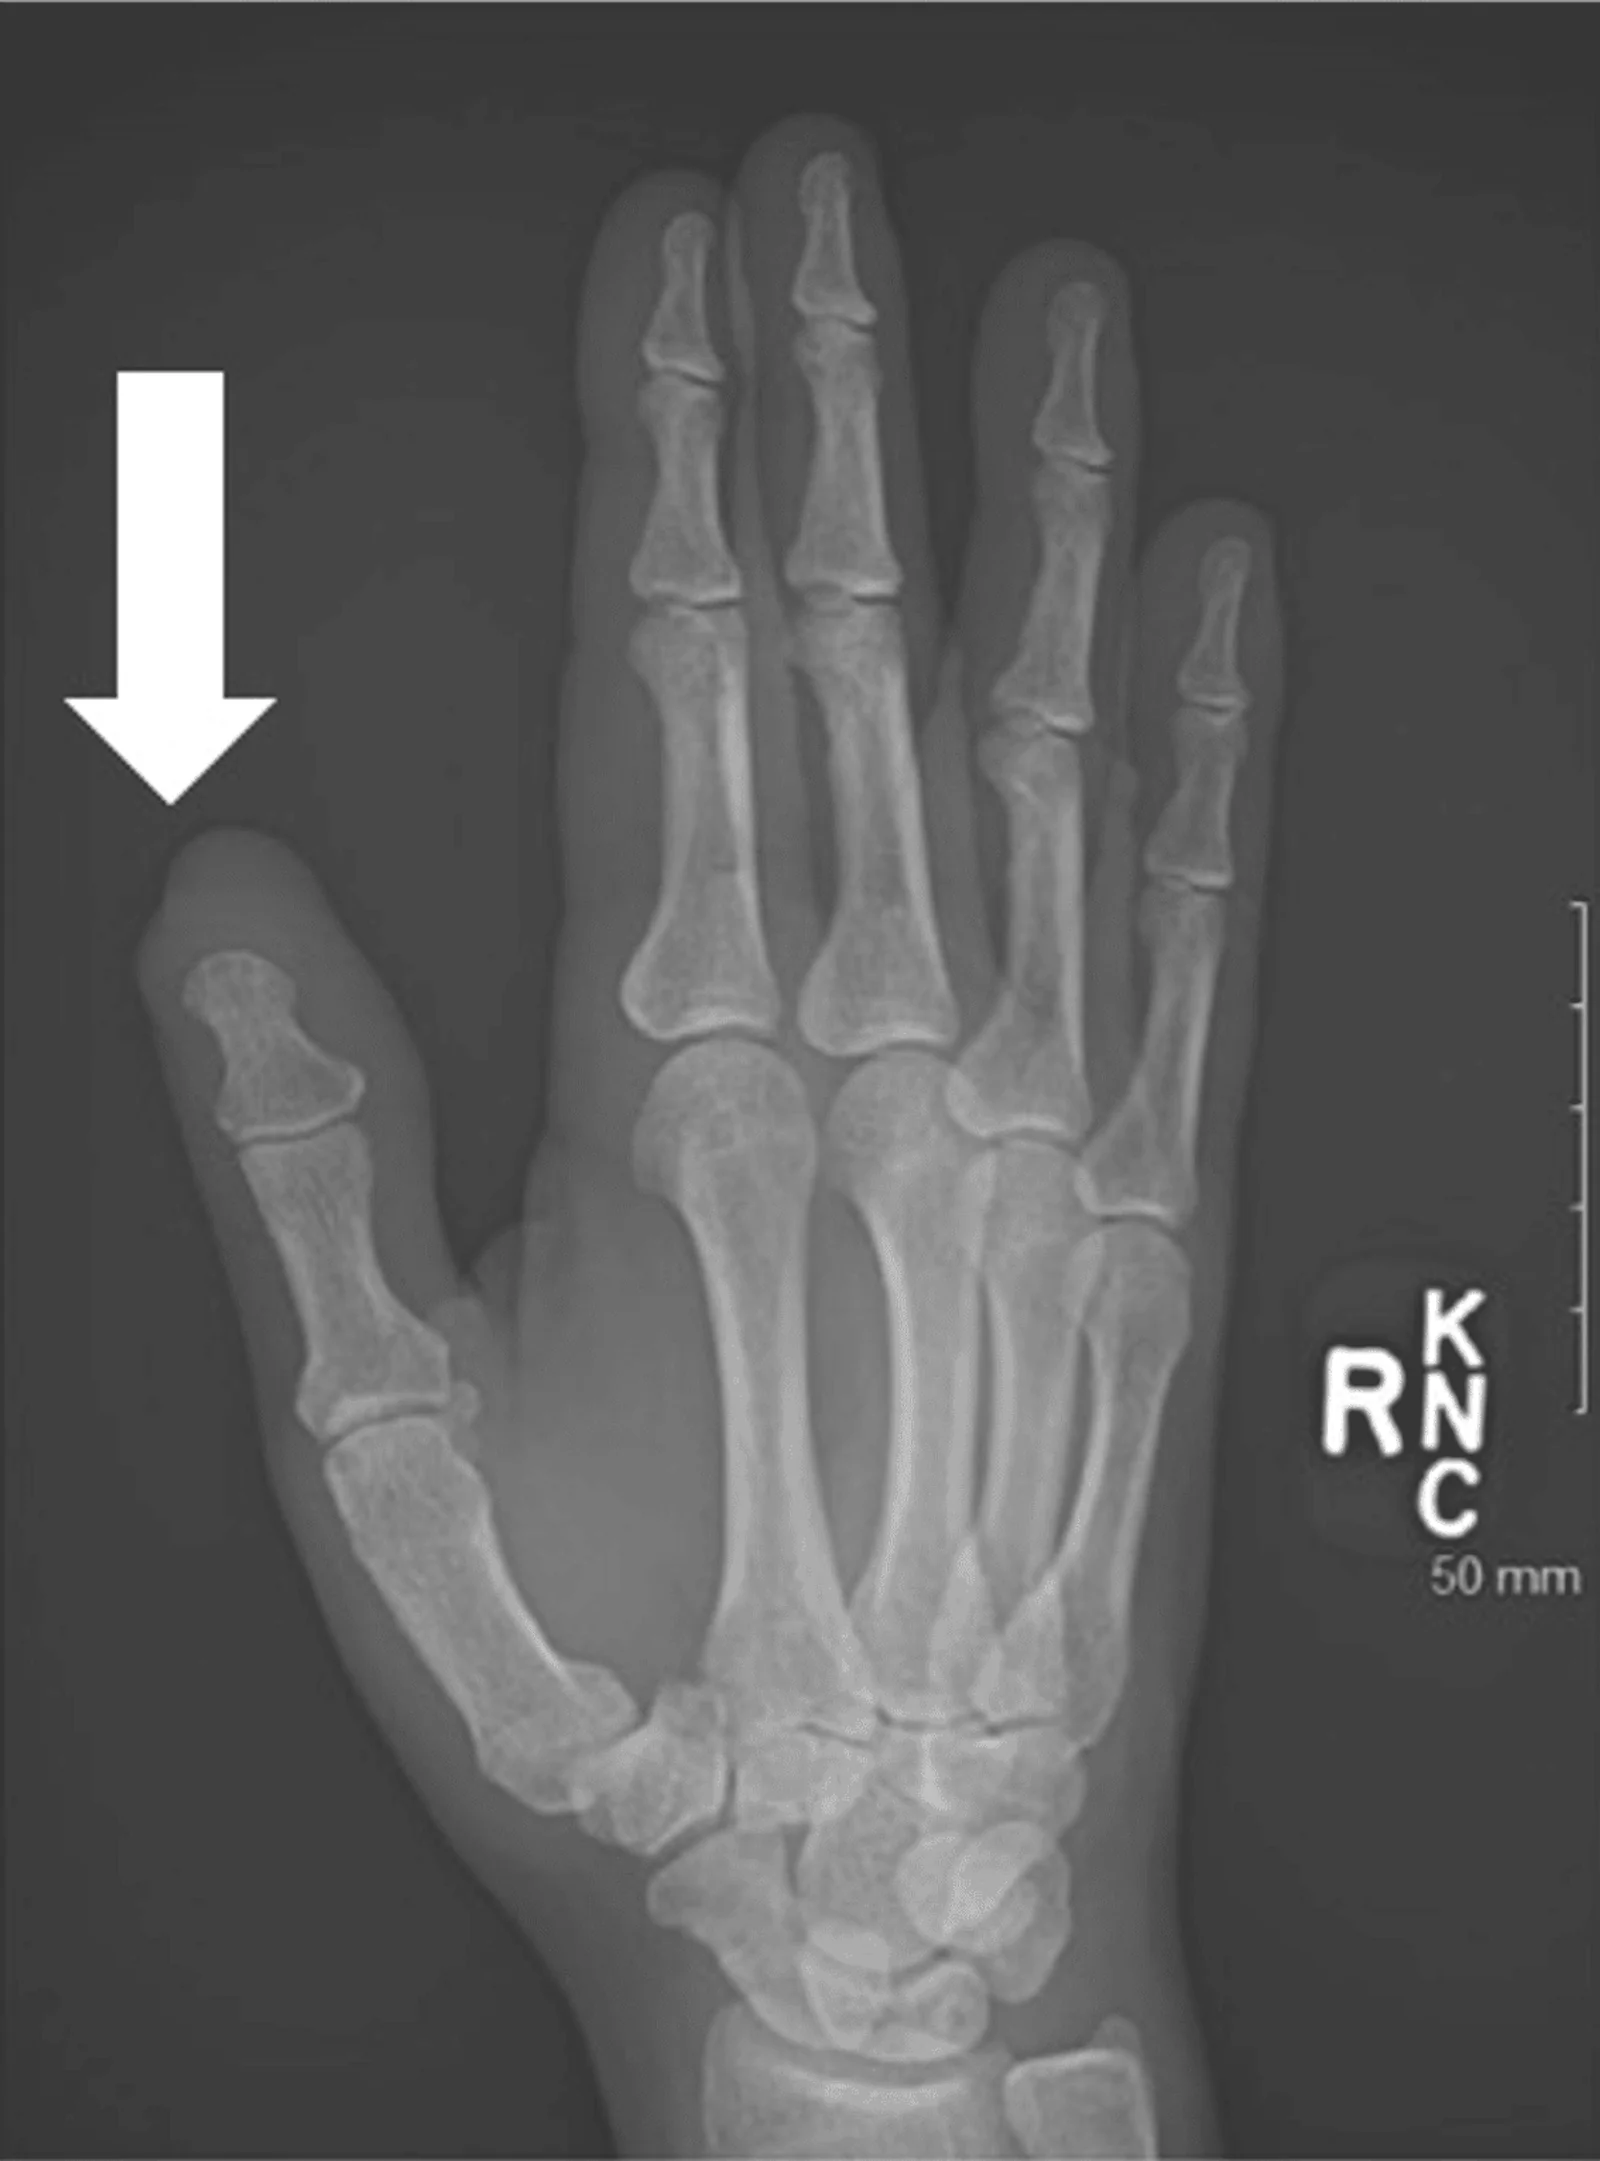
Oblique plain radiograph further delineating the thumb pulp lesion without bony involvement.
